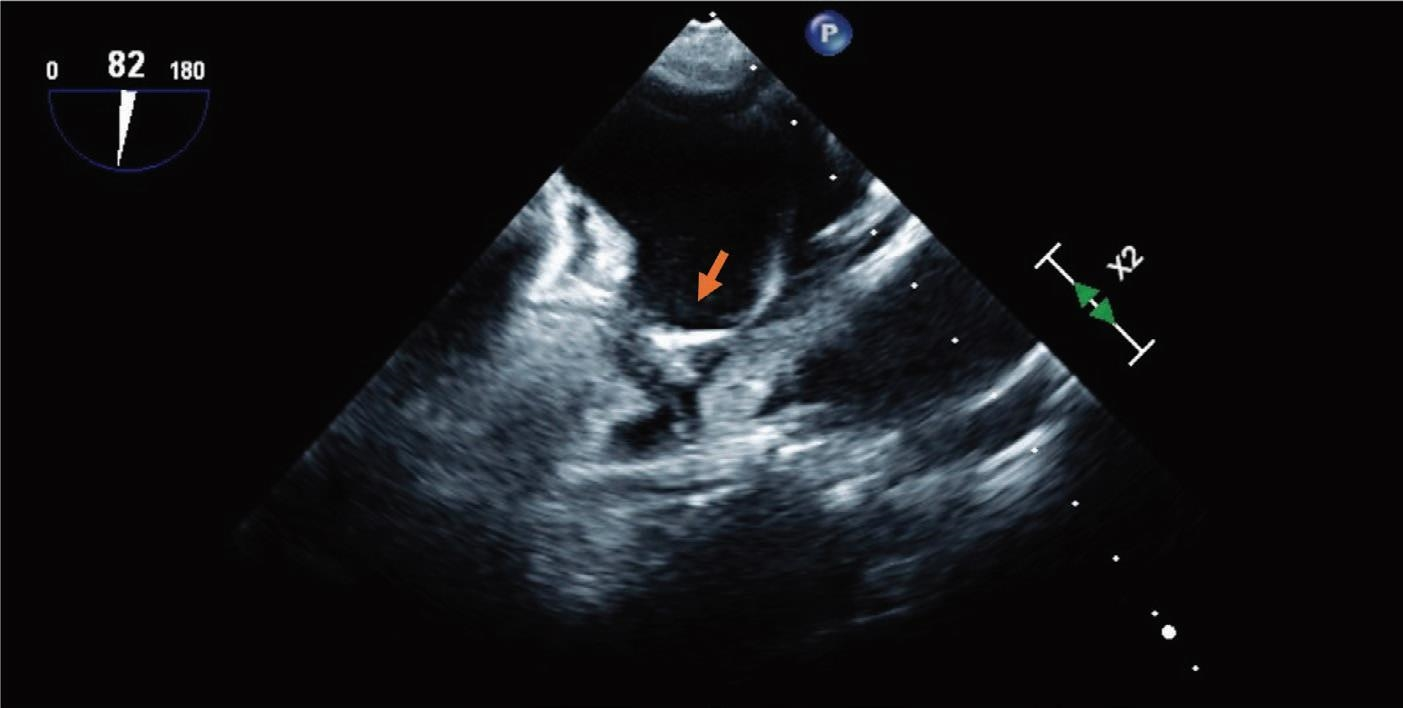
Transesophageal echocardiogram focusing on the left atrial appendage (LAA), showing evidence of thrombus formation.
